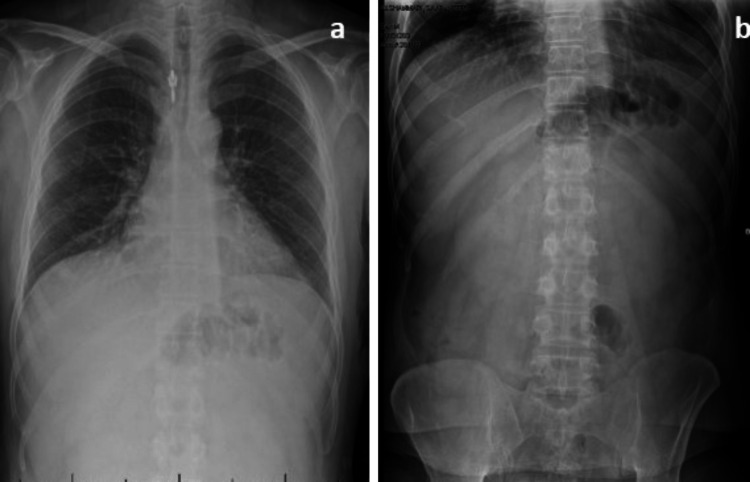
X-ray of the chest in the upright position (a) and X-ray of the abdomen (b) showing no air under the diaphragm.
